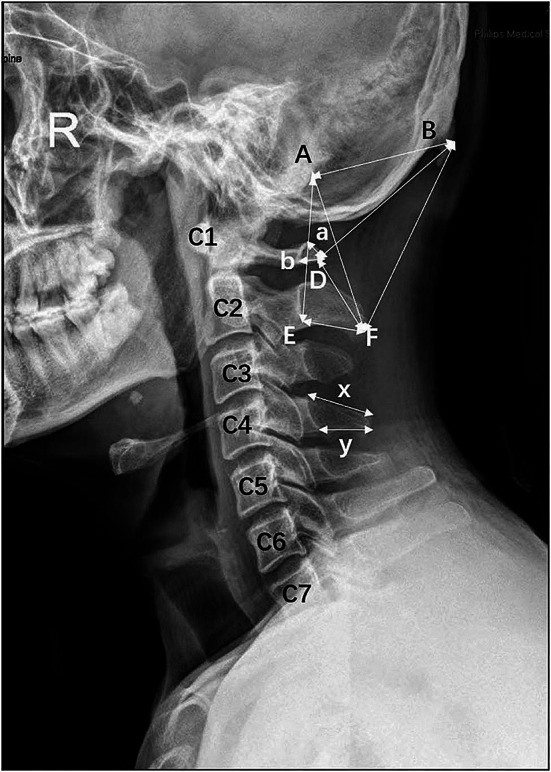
Lateral cervical radiograph. C1- C7 show the line from posterior border of spinal aperture to posterior tubercle of atlas or tip of spinal process; A: the base of the posterior border of the mastoid process; B: The external occipital protuberance; D: the tip of the posterior tubercle of the atlas; E: the anterior end of the inferior border of the spinous process of the axis; F: the tip of the spinous process of the axis; a: the upper border length from the tip of the posterior tubercle of the atlas to the posterior wall of the vertebral foramen; b: the lower border length from the tip of the posterior tubercle of the atlas to the posterior wall of the vertebral foramen; x: the length of the superior border of the spinous process; y: the length of the inferior border of the spinous process
